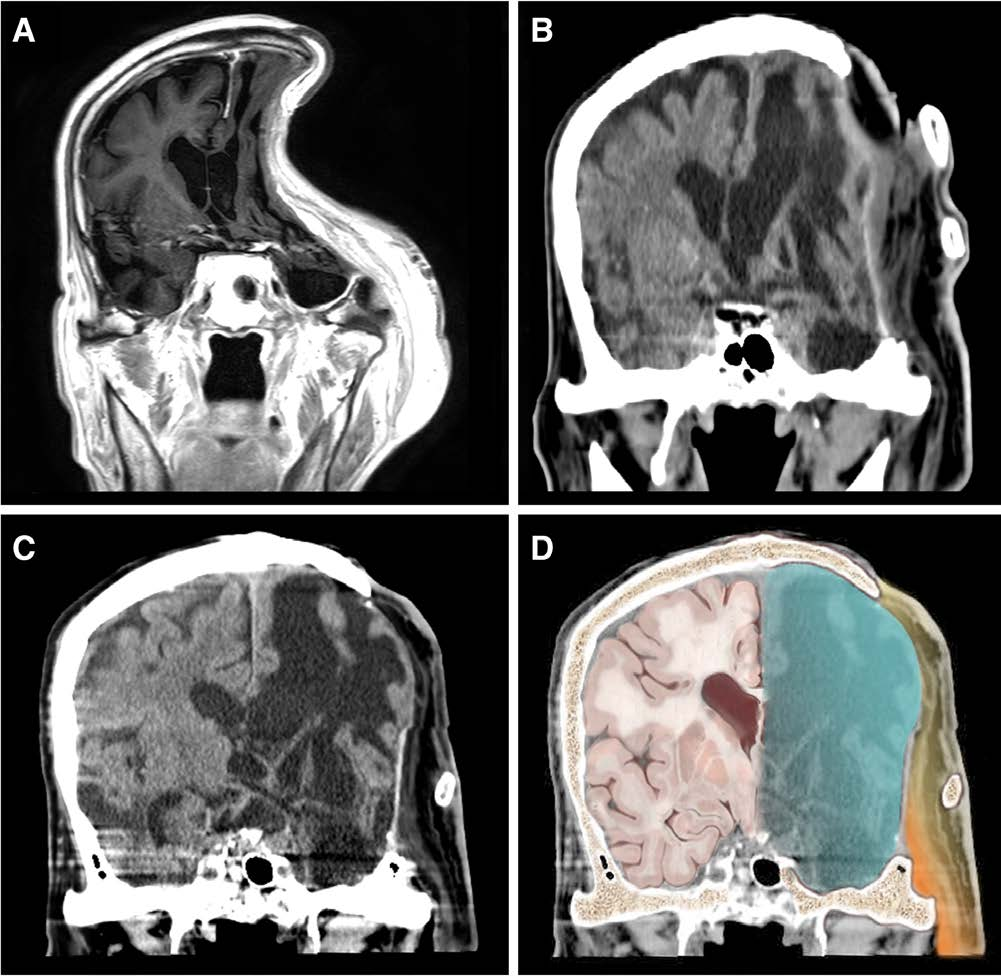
Comparison of coronal views obtained in different stages of the “kebab” technique. (A) Magnetic resonance image 2 months after the first stage of the “kebab” method, showing no fluid accumulation. (B) Nine weeks after the second stage of the “kebab” method. The intracranial volume is shown expanding against the atmospheric pressure after insertion of the rib grafts. (C) Unlabeled image at 2-year follow-up. (D) Labeled image demonstrating the latissimus dorsi myocutaneous flap (*yellow and orange*) and total reversal of sunken skin flap (*blue*).
